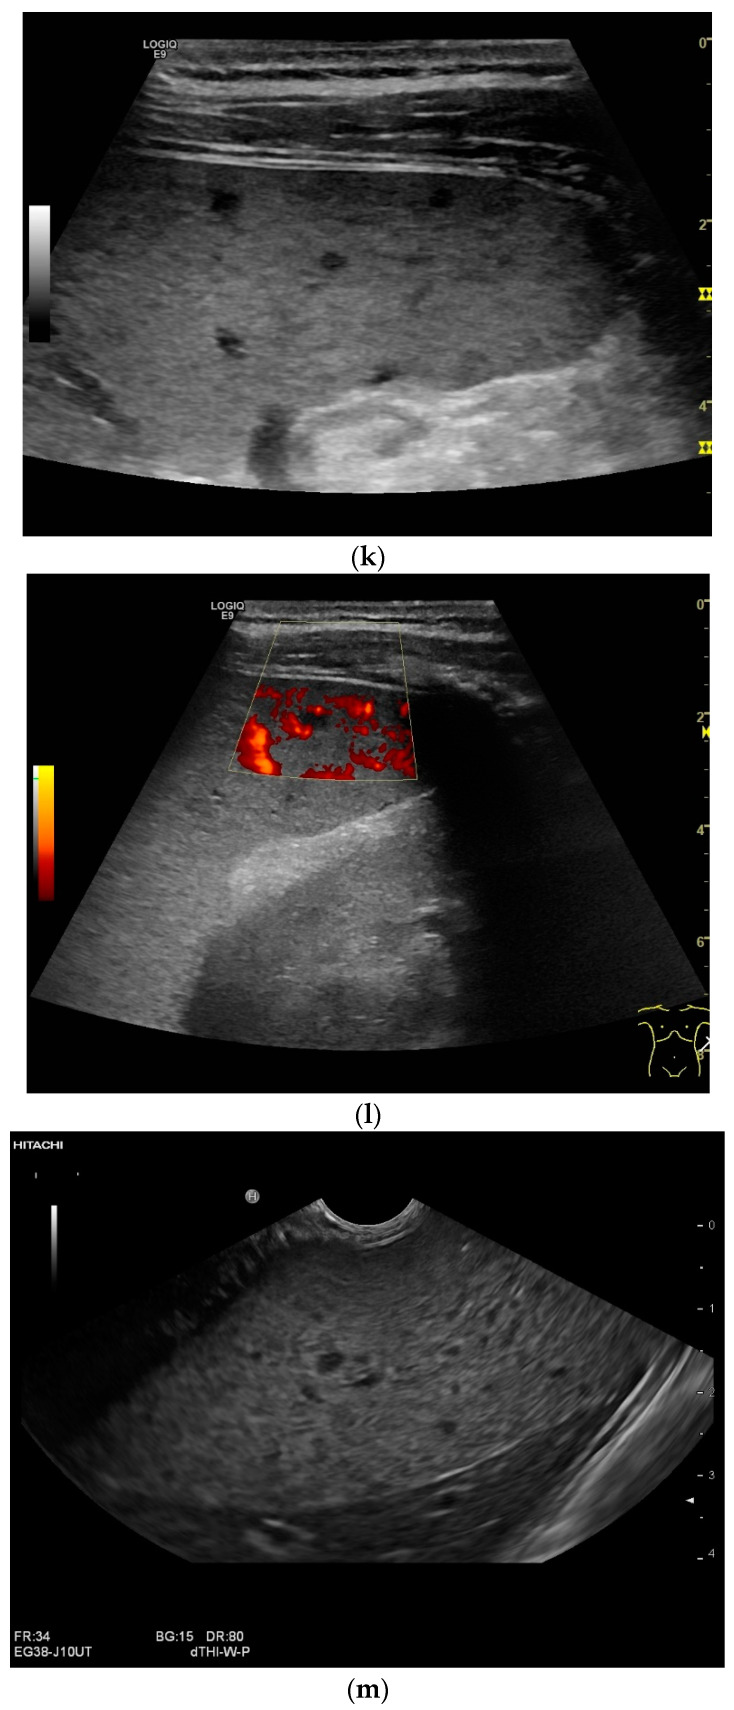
A case of extrapulmonary tuberculosis: 33 y/o male from a country at high risk of tuberculosis. Thoracic pain and fever led to a suspicion of pericarditis. There was no pericardial effusion. There were no pulmonary infiltrations or pleural effusions in the chest CT, but there were enlarged mediastinal lymph nodes. Ultrasound and CT also showed subdiaphragmal enlarged lymph nodes and small nodular splenic changes. The diagnosis was confirmed using the transgastric EUS-guided biopsy (19 G Olympus) of the lymph nodes in the hepatic hilum. Enlarged lymph nodes in the hepatic hilum (a); adjacent to the pancreatic head (b); transabdominal ultrasound (TUS) using a linear transducer of 9 MHz. The hypoechoic central parts are conspicuous (a,b). Rounded lymph nodes are observed as being peripancreatic (c) and as being in close proximity to the gallbladder wall (d). The lymph nodes are visualized between the markers. In the EUS, paragastric lymph nodes are enlarged, rounded, very hypoechoic, with hypoechoic central parts, and forming conglomerates. A central vascular hilum cannot be delineated in the CDI (e). The hypoechoic central parts are softer during elastography (f). EUS also shows enlarged hypoechoic lymph nodes in the hepatic hilus, with hypoechoic central parts that lack a central vascular hilum (g). Elastographically, the lymph nodes are indifferent (h). The diagnosis is confirmed using EUS-guided biopsy (i) with evidence of granulomatous inflammation, caseous necrosis, detection of acid-fast rods, and Mycobacterium tuberculosis in the PCR. In the spleen, single hypoechoic lesions < 5 mm are visible during transabdominal ultrasound using a linear transducer of 9 MHz. Otherwise, fine-grained hypoechoic lesions can only be guessed at (j). With magnification using a 9 MHz transducer, multiple hypoechoic lesions < 3 mm can be delineated (k). These do not reveal any vessels in the Power Doppler (l). EUS confirms multiple hypoechoic splenic lesions, in line with splenic tuberculosis (m).
